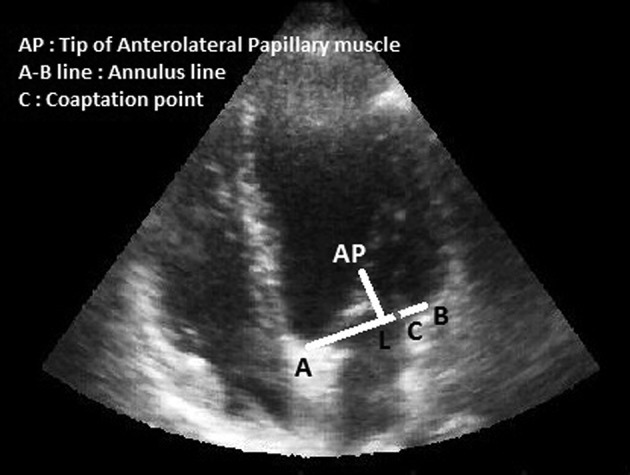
Four-Chamber View in Transthoracic Echocardiography
